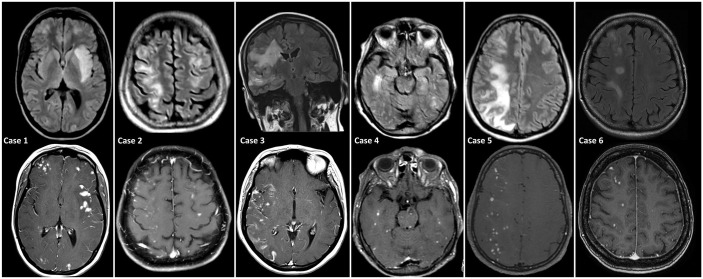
MRI of cases 1 to 5 at initial presentation and follow-up MRI of case 6. The top row shows the T2 FLAIR weighted sequence in each case with partially extensive edema around the lesions. The bottom row shows the typical aspect of NICE lesions after contrast administration with punctate to patchy enhancement in the cortical and subcortical white matter. Case 3 additionally shows cortical gyriform enhancement due to a contemporaneous ischemic stroke.
